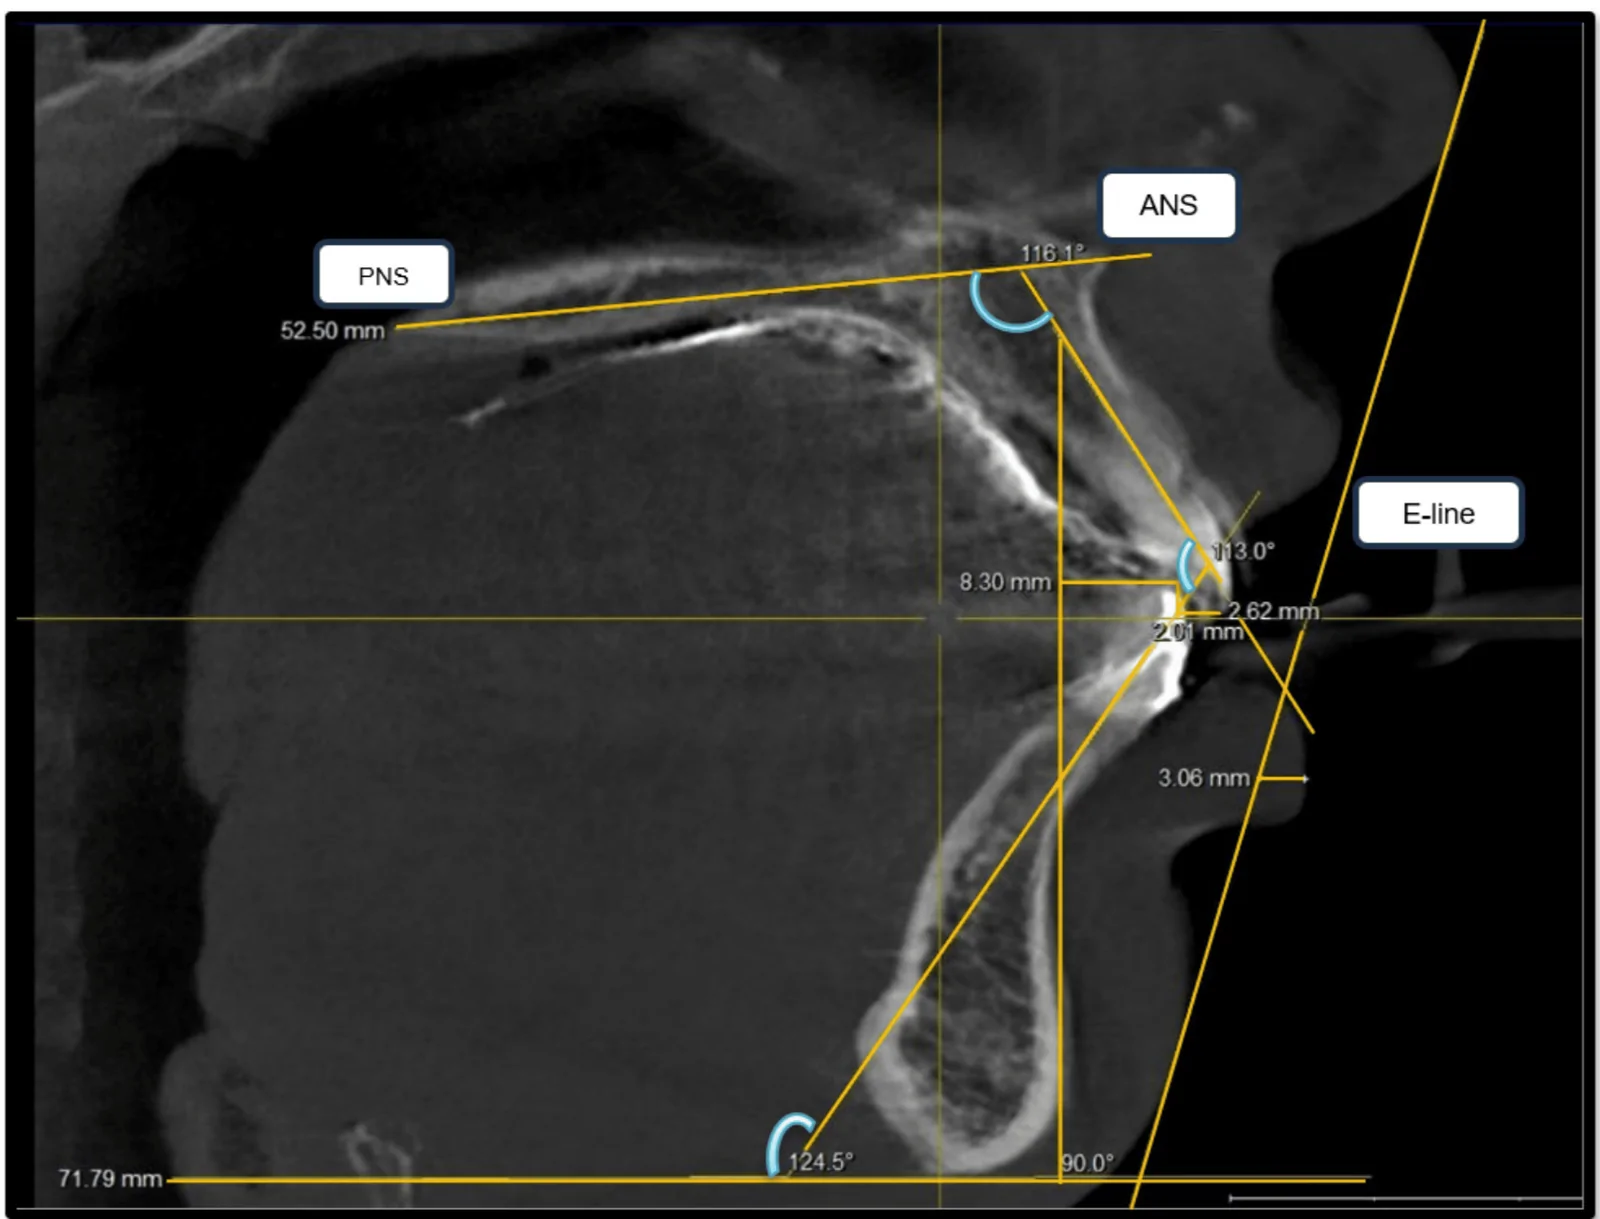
Dento-alveolar angular and linear measurements on CBCT The figure shows the angular measurements measured on the CBCT, such as U1-PP, L1-MP, and interincisal angle, as well as the linear measurements such as tongue tip position, overjet, overbite, and lower lip retraction. ANS - anterior nasal spine; CBCT - cone beam computed tomography; E-line - esthetic line; L1-MP - inclination of lower central incisor to mandibular plane (horizontal reference plane); PNS - posterior nasal spine; U1-PP - inclination of upper central incisor to palatal plane (anterior nasal spine to posterior nasal spine)
